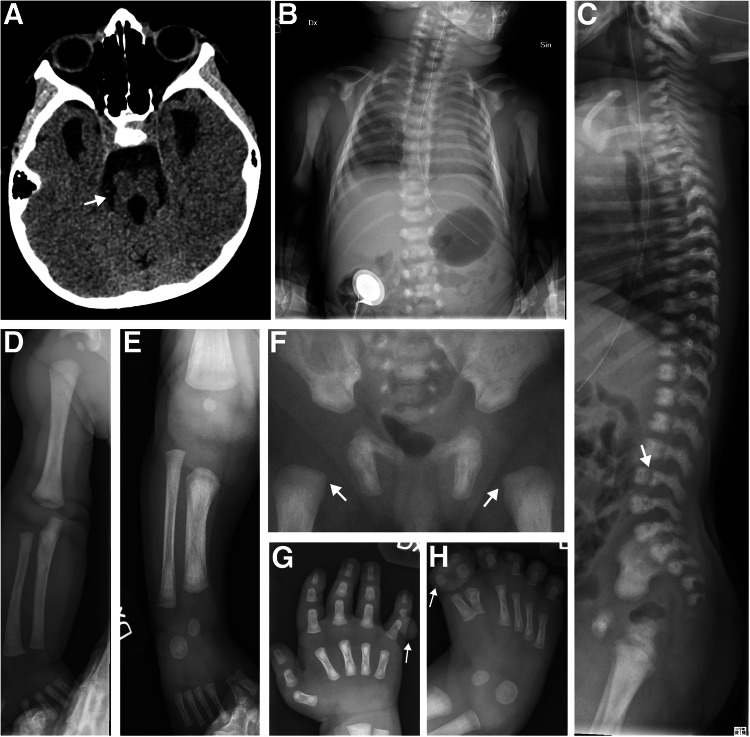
Skeletal radiographs of patient 28 with a clinical diagnosis of orofaciodigital syndrome. CT at 3 years and radiographs at 1 month of age. A Cerebral CT, showing molar tooth sign (arrow). B Chest, thoracic cage is within normal range. C Lateral spine, with normal height of vertebral bodies. Note sporadic coronal clefts (arrow). D Right upper extremity, normal. E Lower right extremity shows short tibia and broad diaphyses. Proximal tibial epiphysis is not ossified. F Pelvis, normal. Note short femoral necks (arrows). G Right hand with a rudimental postaxial extra finger (arrow). H Foot, note preaxial polydactyly (arrow).
